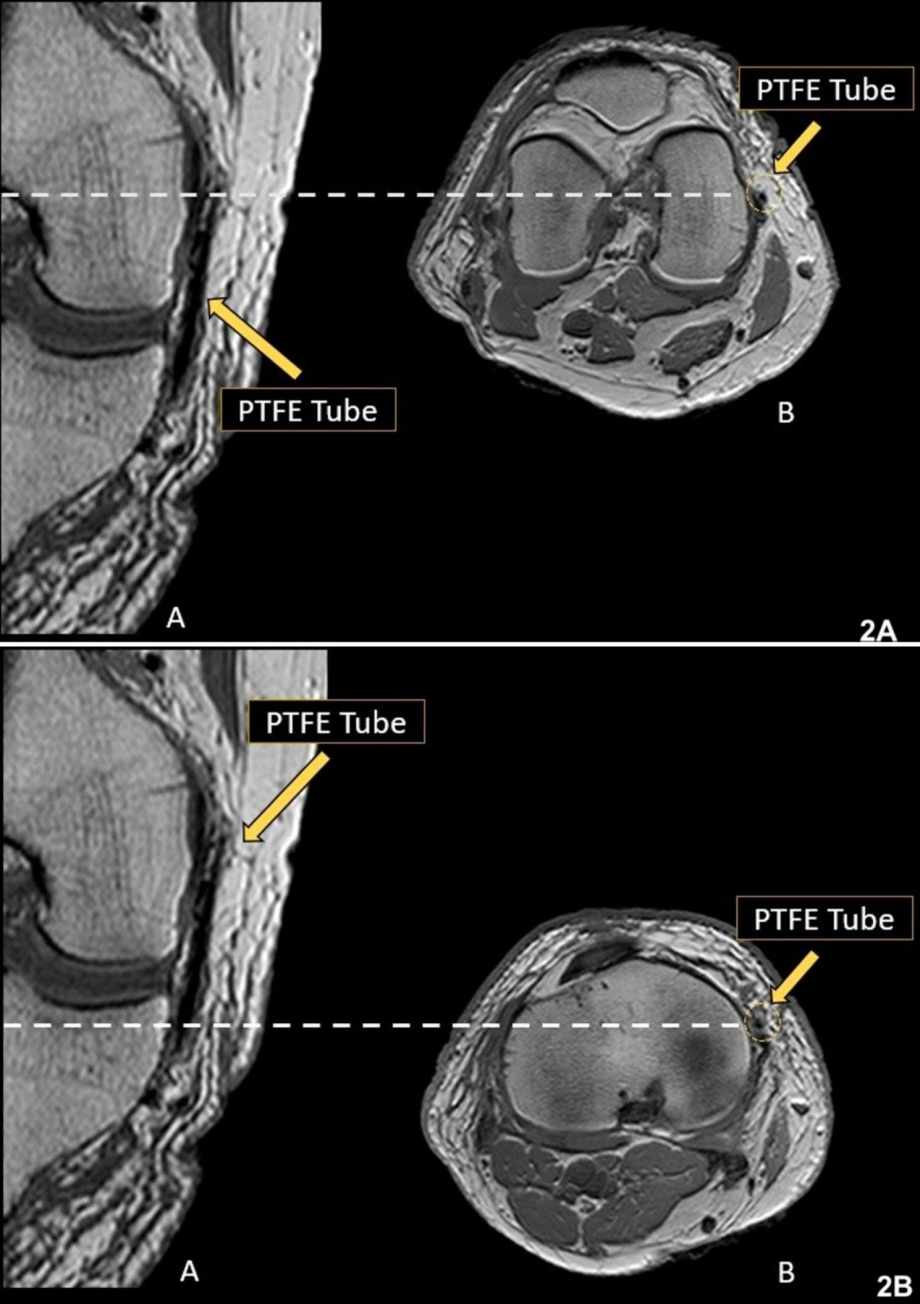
MRI scans with PTFE tube in the AOL proximal (2A) and distal (2B) topography
